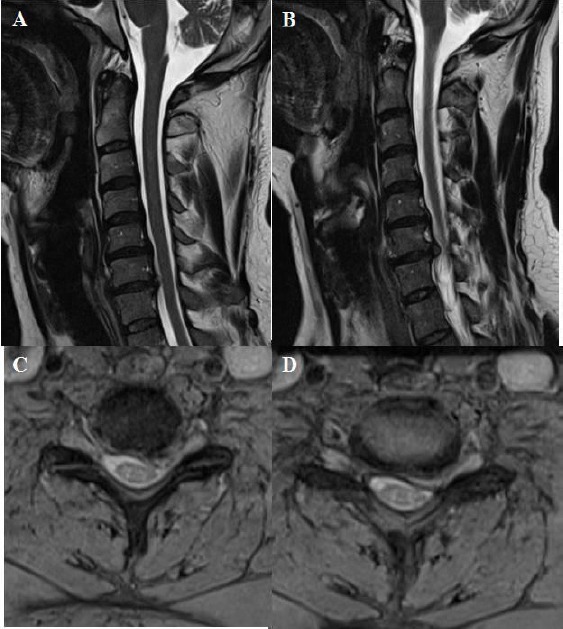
A, B, C, D: T2 weighted sagittal and axial MRI showing one level cervical root compression due to soft cervical disc extrusion on the left side between cervical 6 and cervical 7. In addition, cervical lordosis angle was measured 22.8 degrees on lateral X-ray at the preoperative period*.
